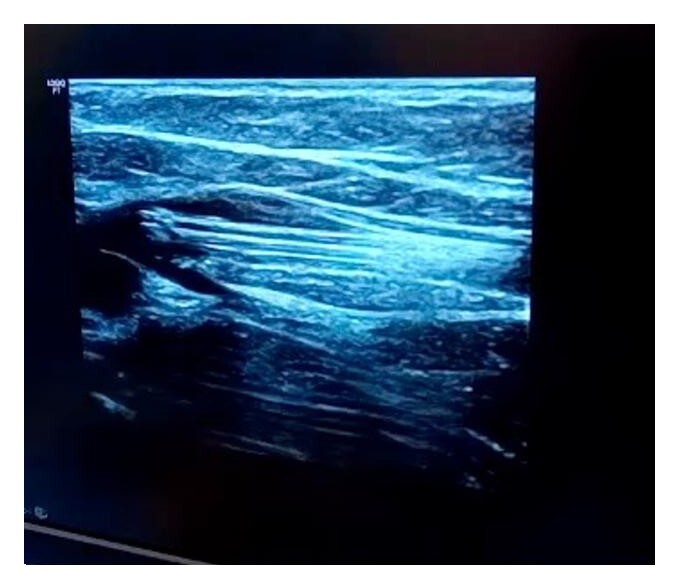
Intraoperative ultrasound image showing the Flebogrif® catheter with hooks open at the level of the saphenofemoral junction.
